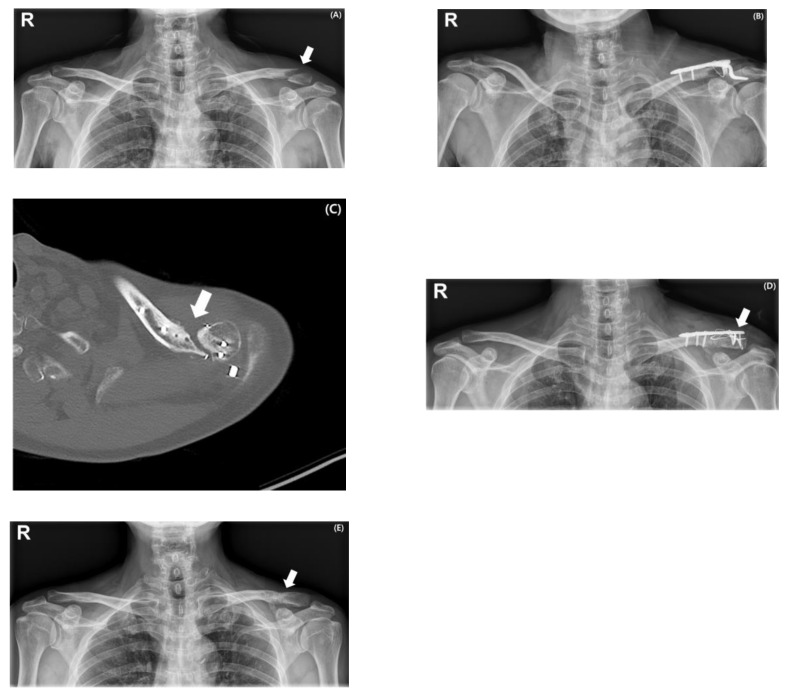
Serial radiographs and an axial CT image of a 59-year-old man with a displaced distal clavicle fracture (Type IIB) following a fall from height (indicated by a white arrow) (A). Initial treatment involved hook plate fixation with additional cerclage wiring (B). At 3 months postoperatively, an axial CT image demonstrated a persistent fracture gap prior to planned implant removal (indicated by a white arrow) (C). Revision surgery was performed using a distal clavicle locking plate with cerclage wiring and tension band wiring (indicated by a white arrow) (D). Radiograph obtained at 3 years post-revision confirmed complete fracture union, following implant removal, which had been performed at 1 year post-revision (indicated by a white arrow) (E).
